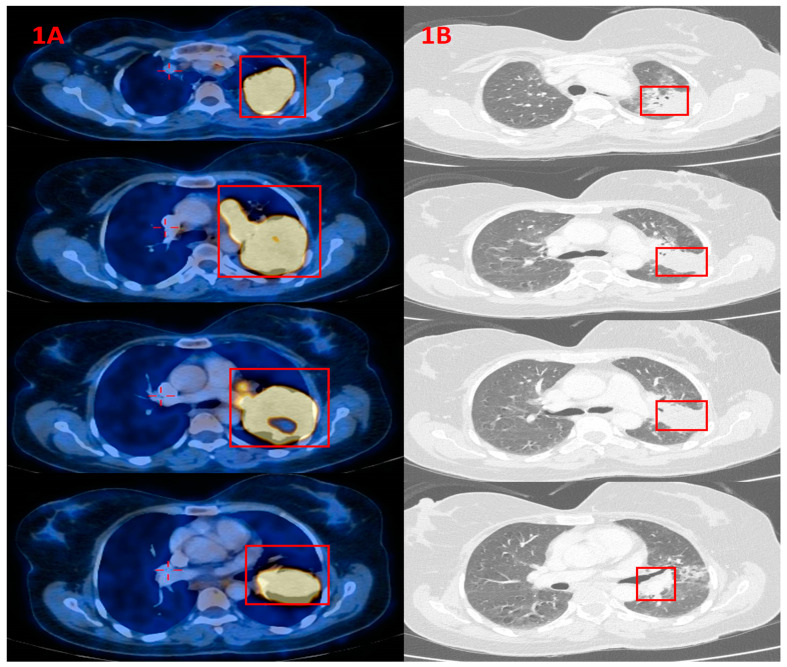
(A) PET-CT (29 April 2021) with hypermetabolic uptake of the tumor in the left lung (red squares), before starting alectinib. (B). CT scan of chest (12 July 2021) showing radiological significant improvement, with the left lung mass having decreased in diameter (red squares) after starting alectinib, along with elevation and subsequent normalization of ALP values.
